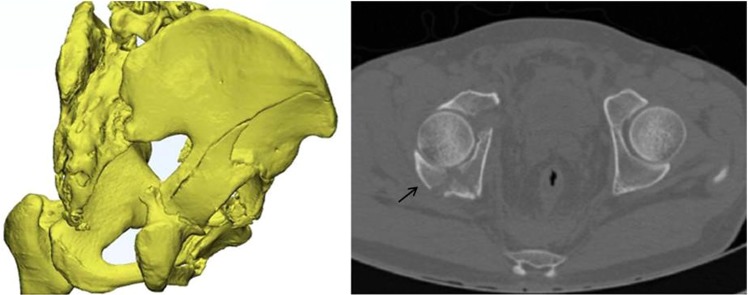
CT reconstruction and axial section images showing the posterior wall fragment associated with a both-column acetabular fracture.
